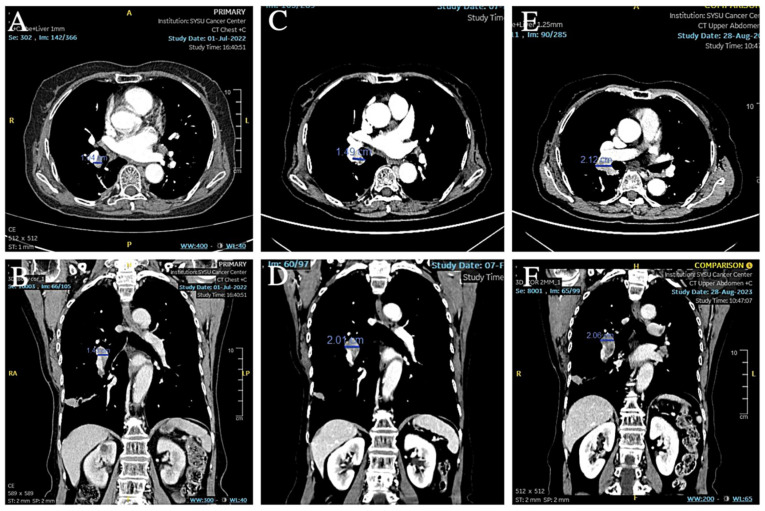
(A,B) CT showed that the short diameter of the right hilar lymph node had increased to 14 mm. (C–F) CT showed that the right hilar lymph node continued to increase in size and the short diameter increased to 21 mm.
